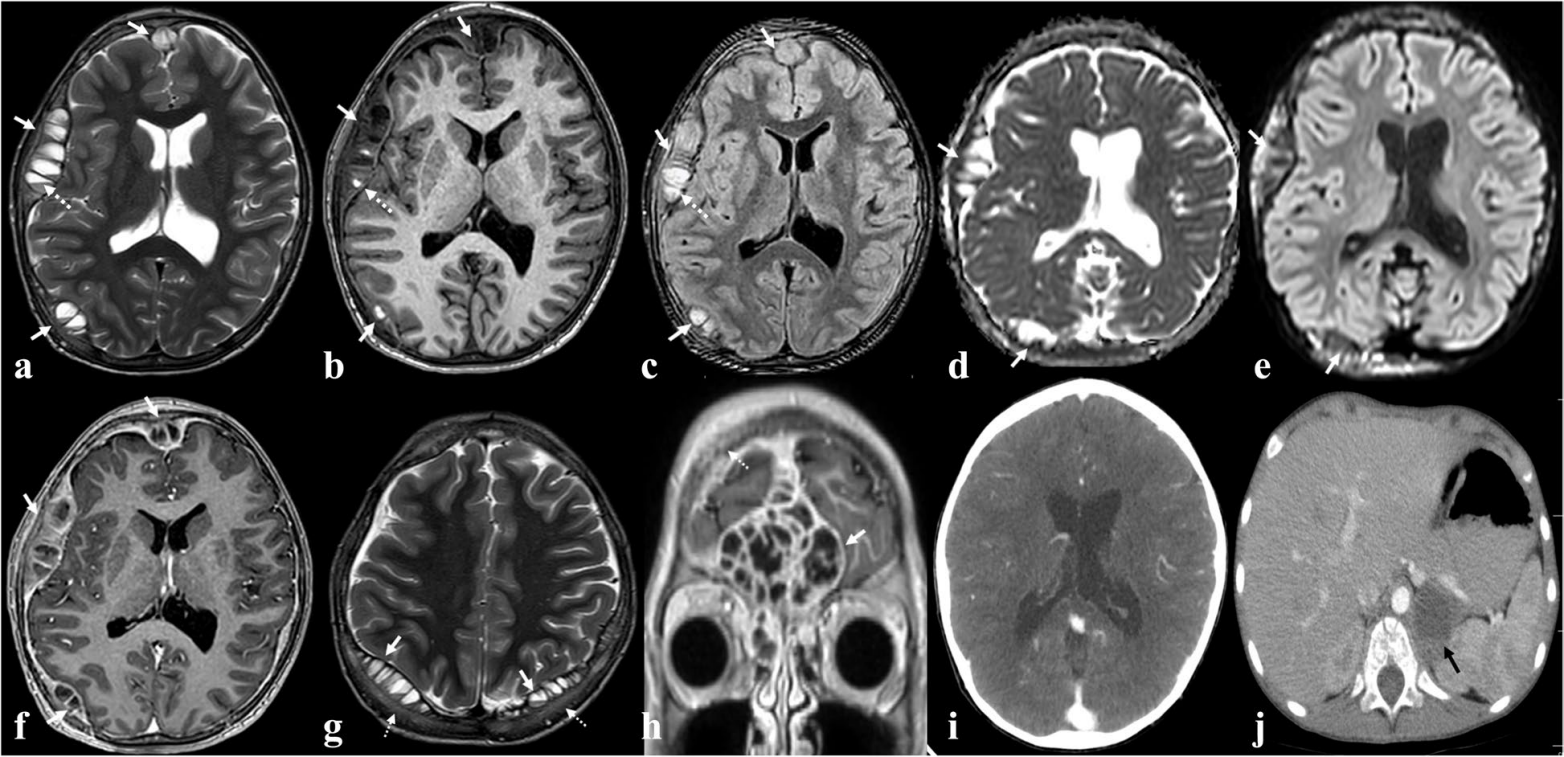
Brain MRI with and without contrast media administration [a to h] revealed multifocal multi-loculated cystic lesions in the epidural space [white arrows in all images] containing fluid–fluid levels [dashed white arrows in a, b and c] which the dependent fluid was hyperintense on axial T1w and FLAIR [b and c respectively] and hypointense on axial T2w [A] sequences compatible with blood representing blood-fluid level. Diffuse pachymeningeal enhancement and enhancement of wall and septa of lesions are noted on axial contrast-enhanced T1w sequence [f]. Image [g] shows involvement of overlying calvarial bones as hyperintense signal change on axial T2 w sequence [dashed white arrows]. DWI and ADC map sequences [e and d] show no restriction. Image [h] is coronal contrast-enhanced T1w sequences showing similar large epidural metastatic lesion around flax [white arrow], note the calvarial involvement of frontal bone as abnormal bone and adjacent dural enhancement with overlying enhancing soft tissue component [dashed white arrow]. Follow up CT scan after chemotherapy treatment [i] shows complete resolution of meningeal lesions. Image j shows low attenuating soft tissue mass in left adrenal gland [black arrow]
